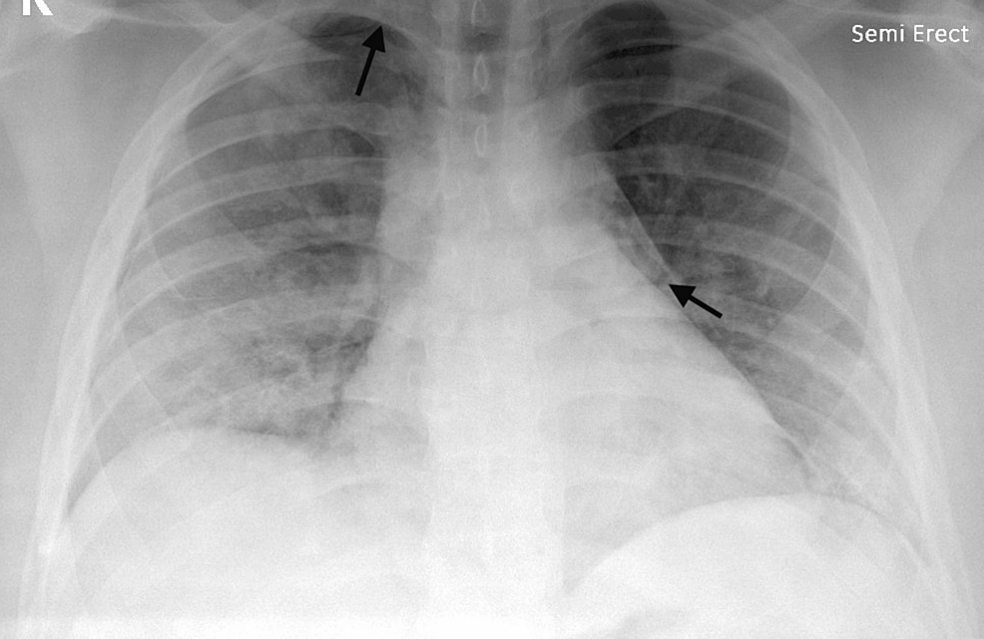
Case 5 chest X-ray Arrows indicating pneumothorax on the right side of the chest and pneumomediastinum on the left side of the chest
